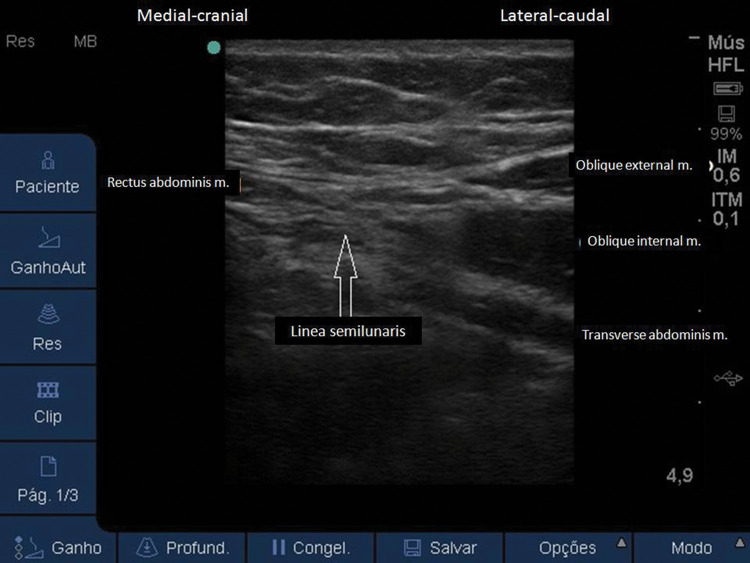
Ultrasound image with visualization of the lateral portion of the RA muscle, linea semilunaris and abdominal muscles planes laterally.
